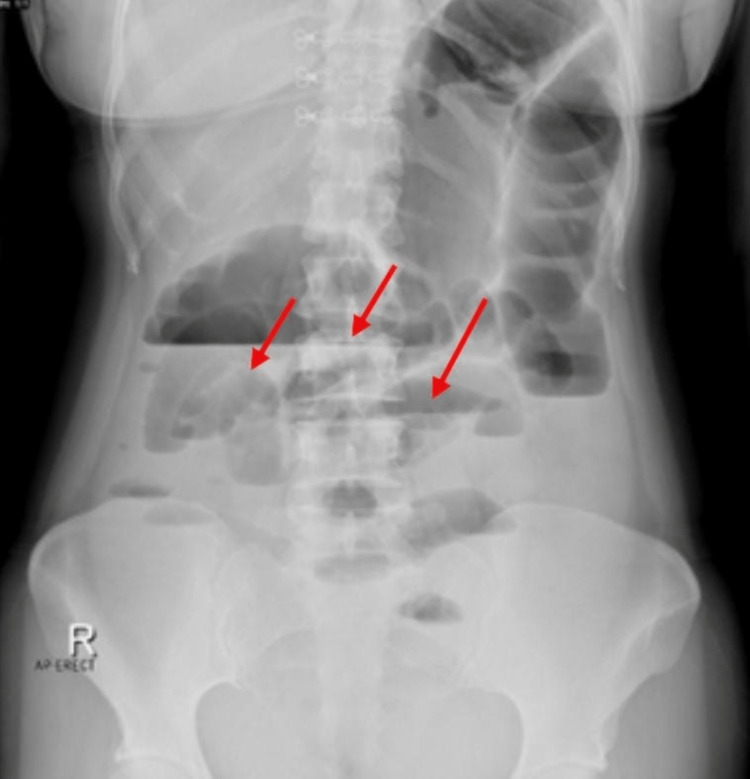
Abdominal X-ray showing dilatation of large and small bowel loops (red arrows).
